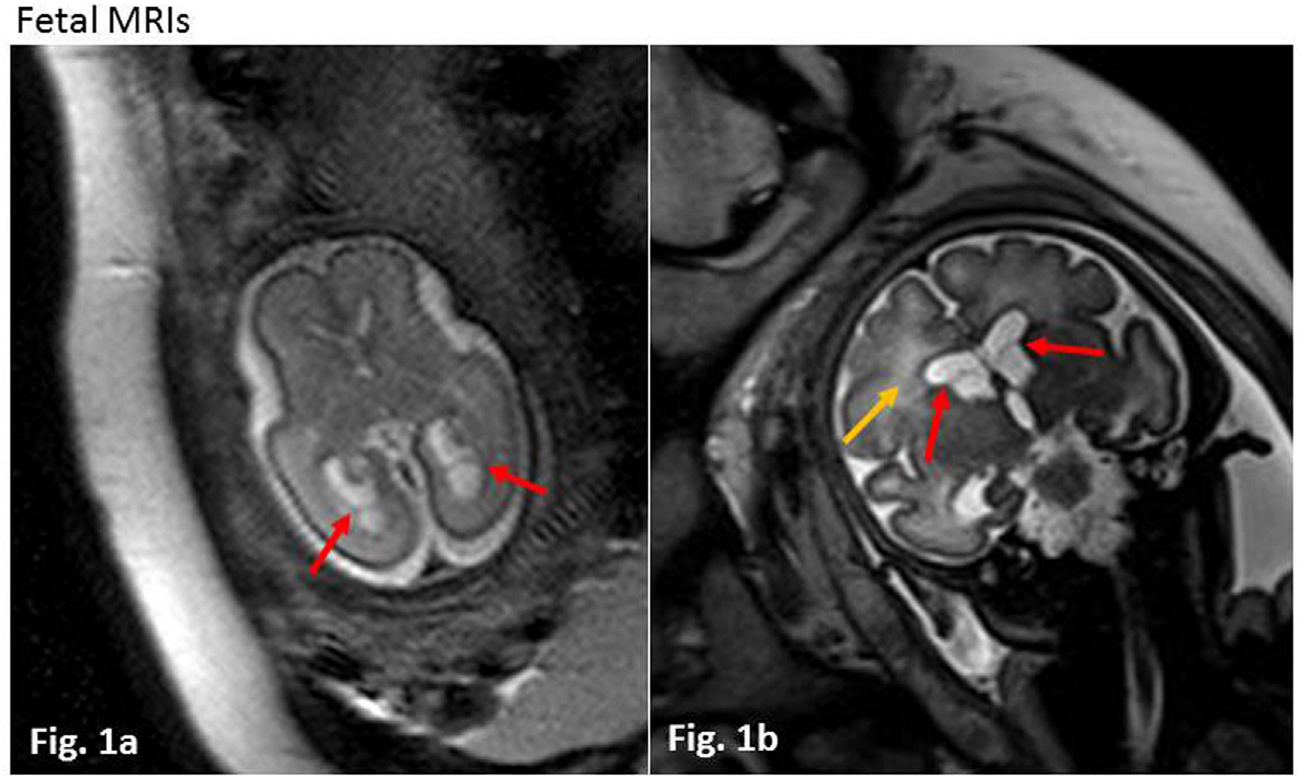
Fetal magnetic resonance imagings (MRIs). (A) Week 26 of gestation, axial T2 haste weighted sequence. Red arrows indicate intraventricular cysts in the posterior horns of slightly dilated lateral ventricles. (B) Week 36 of gestation, coronar T2 haste weighted sequence. Red arrows show intraventricular cysts, yellow arrow marks diffuse white matter lesions with increased T2 intensity.
